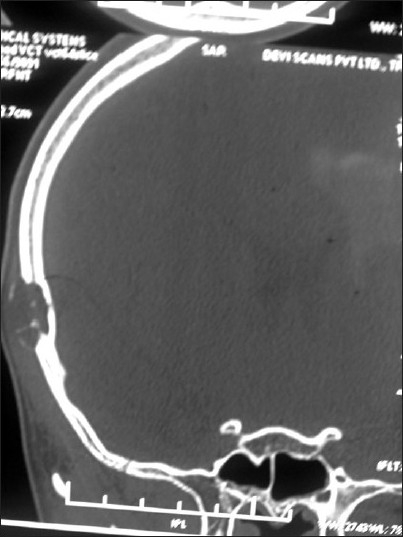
Plain CT head, coronal section, shows a focal osteolytic lesion in the right high temporal region, with interruption of the outer table and an intact inner table of the skull
